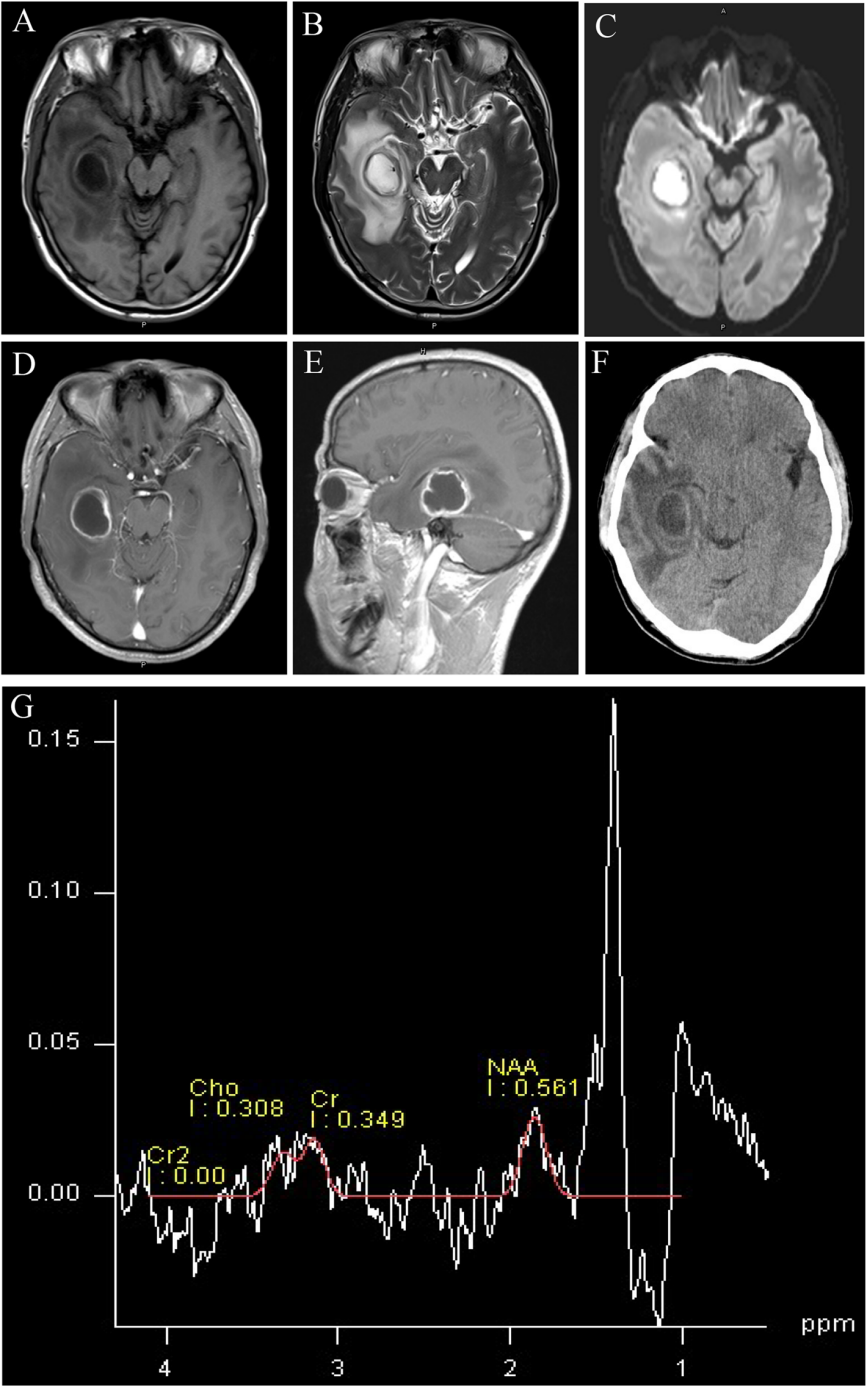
Head MRI and CT images prior to the first surgery. a-b A quasi-circular space-occupying lesion was found with long T1-weighted and T2-weighted signal shadows in the right temporal lobe and was surrounded by large patches of edema. c Enhanced T1-weighted FLAIR signal were observed in the center of lesion. d-e The signal intensity of the ring wall was increased after enhancement. f CT scan showed a circular space-occupying lesion with slightly high density ring wall and low density shadows in the right temporal lobe that was surrounded by large patches of edema. g MRS showed an elevated lactic acid peak, and low NAA, choline, and creatine peaks
